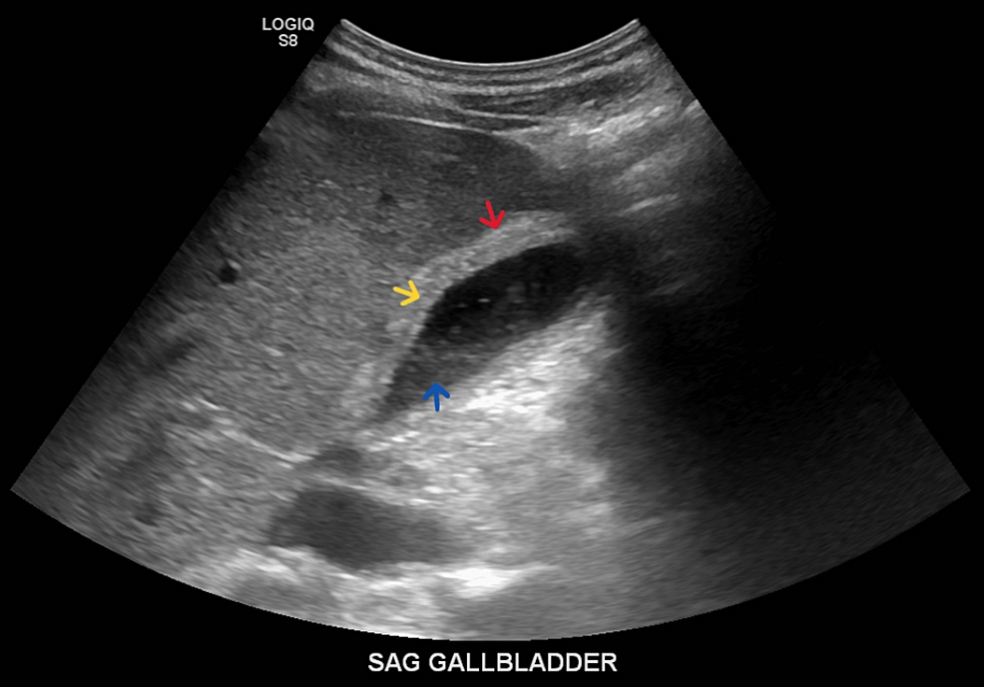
Right upper quadrant abdominal ultrasound showing gallbladder containing sludge (blue arrow), with gallbladder wall thickening (red arrow) and pericholecystic fluid (yellow arrow), suggestive of acute cholecystitis.
